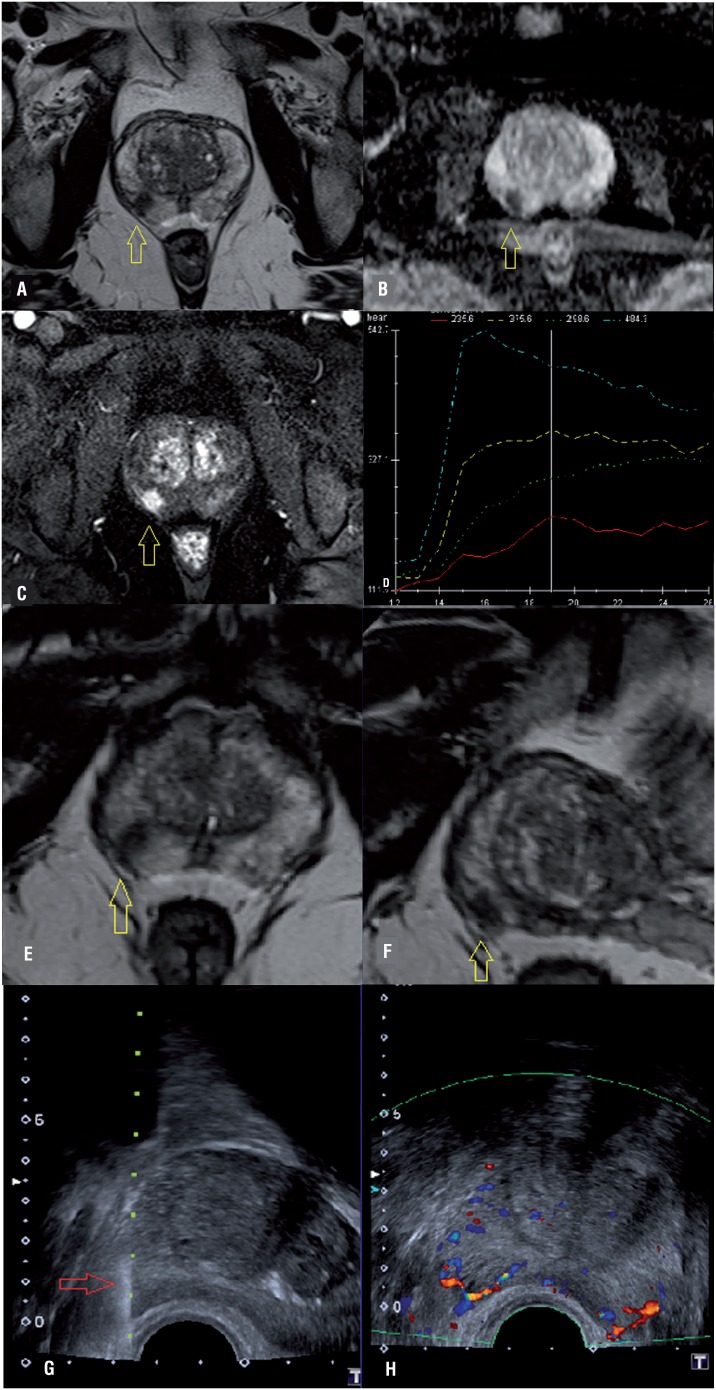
MpMRI example of a suspicion lesion on the right lobe of peripheral zone of the prostate: A) a round lesion on T2 weighted-image; B) ADC map shows diffusion restriction; C) dynamic-contrast-imaging with hypervascularization and D) washout (blue line). Patient received US/MRI-guided biopsy where we can see the lesion on MRI (E and F) and in real time on US (G), helping to target the lesion (H).
